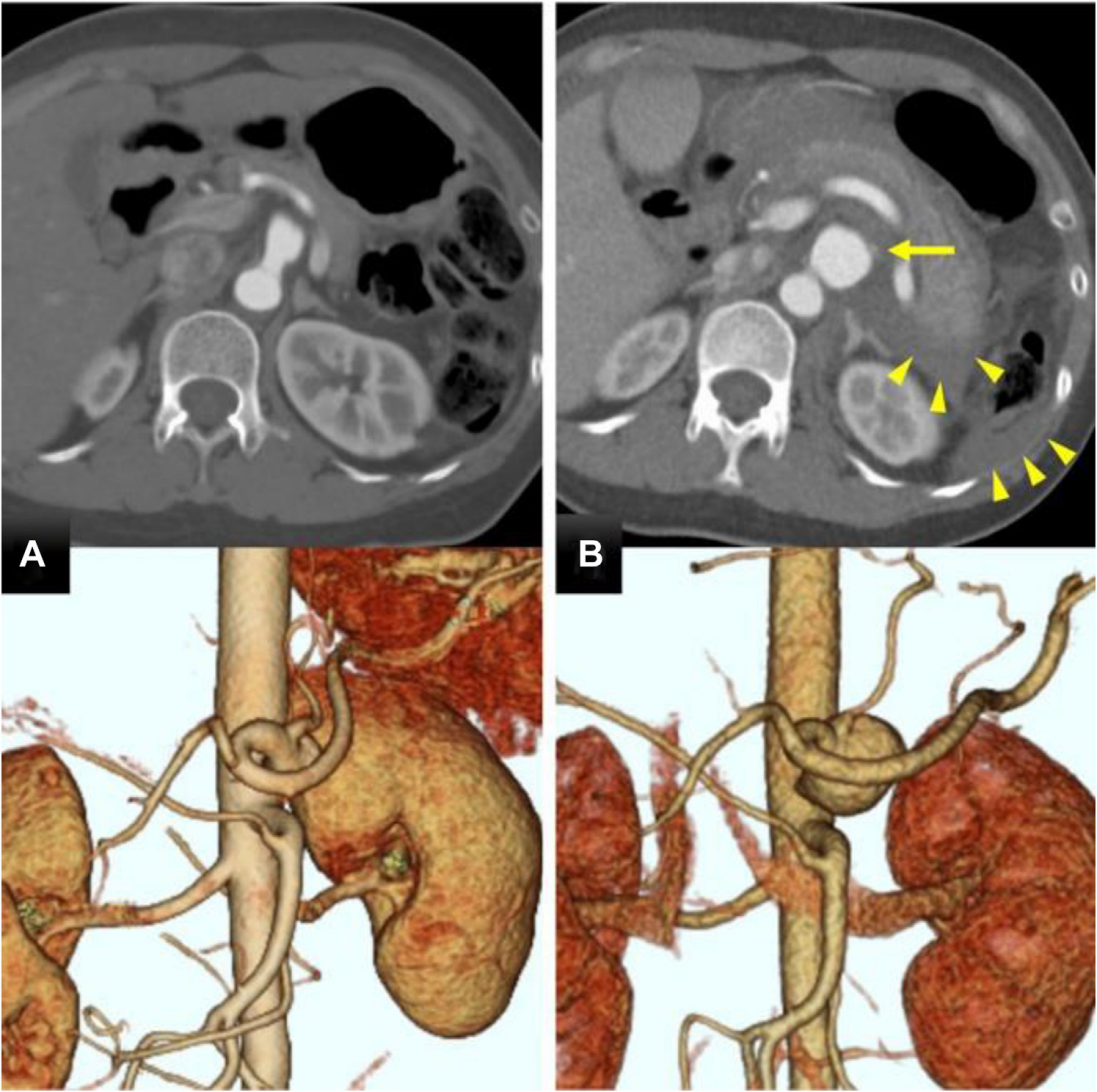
A, Computed tomography (CT) scan revealed a celiac artery aneurysm (CAA) with a diameter of 14 mm, immediately after the aortic bifurcation. B, The aneurysm expanded to 26 mm in just 1 day, and extravasation *(arrow)* and intra-abdominal fluid accumulation *(arrowheads)* were observed.
